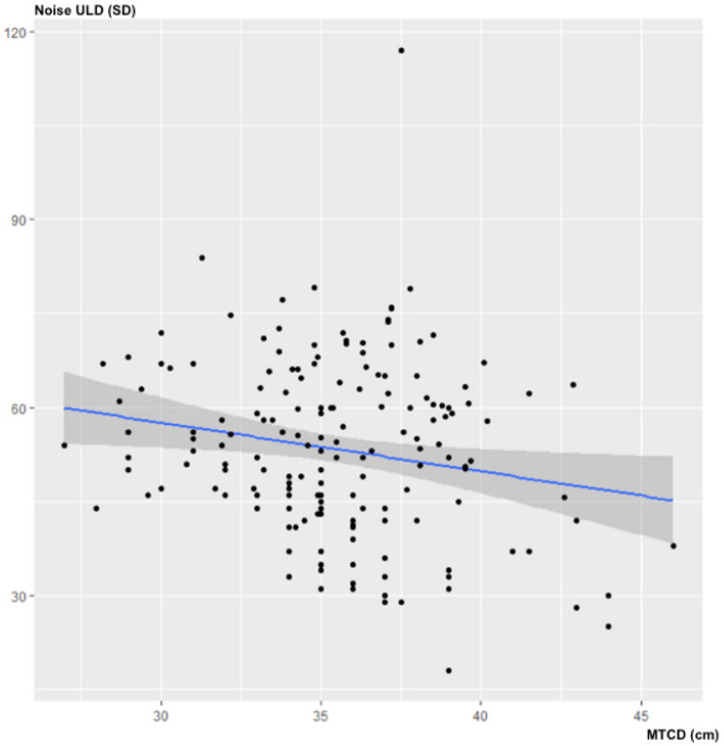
Influence of MTCD on image noise (in SD) for ULD-CT.
